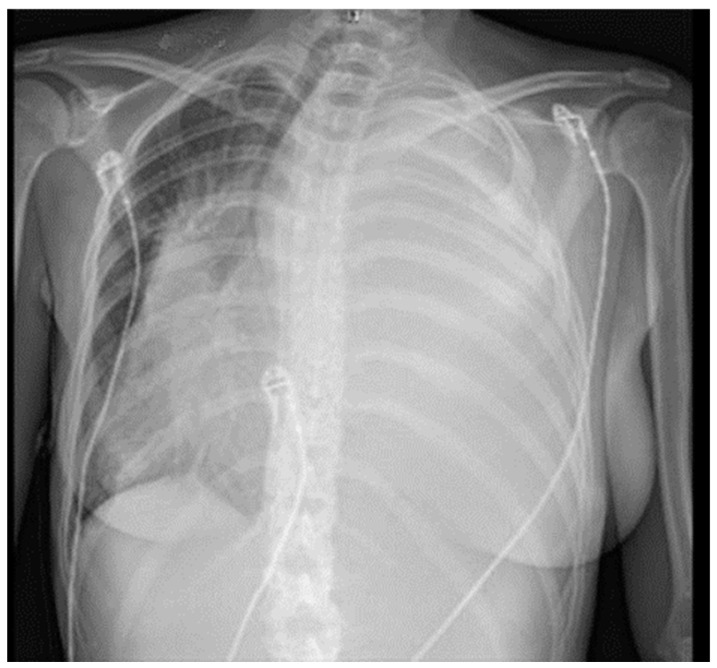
Chest X-ray at presentation.
